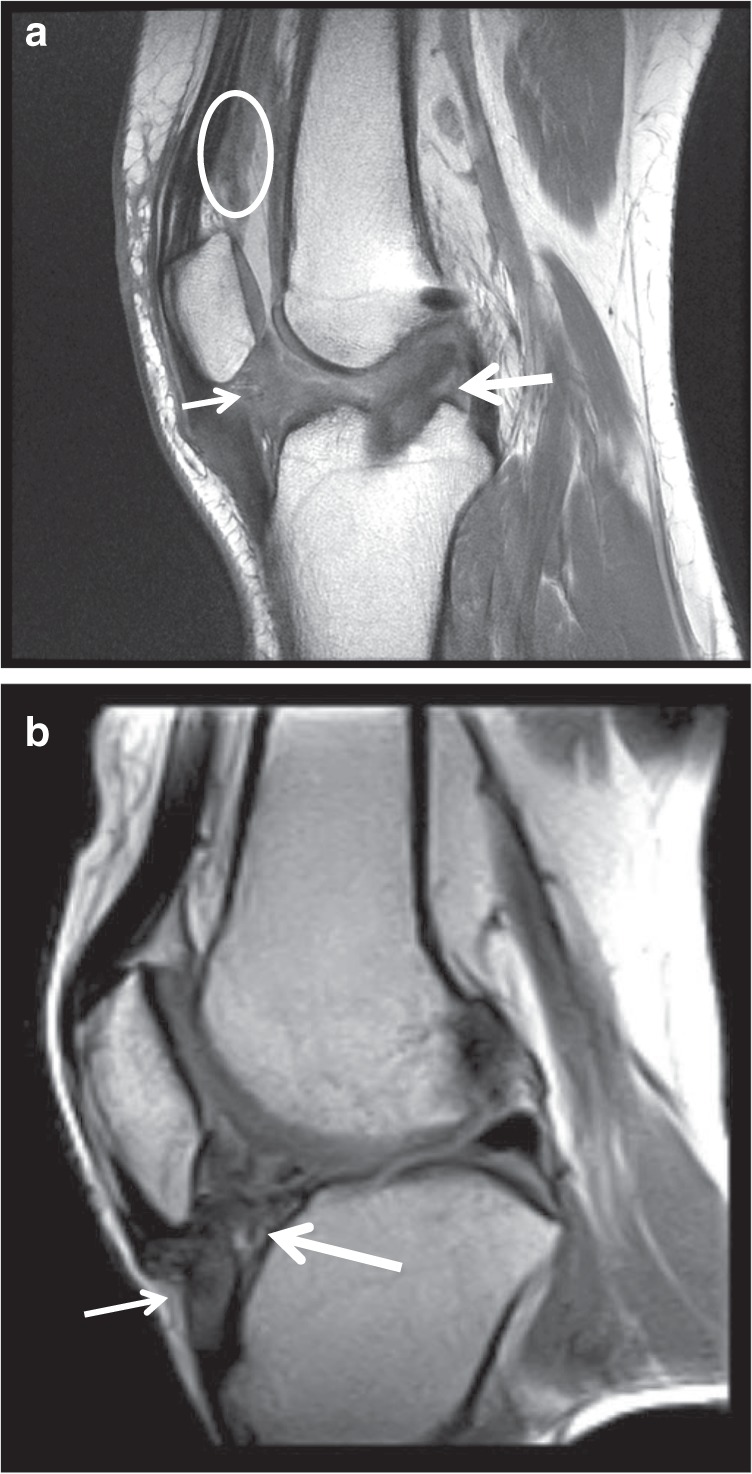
a Sagittal fast spin echo intermediate-weighted image of a 33-year-old woman with clinical stiffness following anterior cruciate ligament (ACL) reconstruction, showing scarring of the synovium around the ACL reconstruction (long arrow) as well as the central portion of the deep infrapatellar fat pad (short arrow) and the lining of the suprapatellar recess (oval). b Magnetic resonance imaging of the knee of a 49-year-old male with clinical stiffness 2 months following a meniscus operation, showing deep infrapatellar fat pad scarring (long arrow) and shortening of the patella tendon (short arrow) with resultant patella infera (abnormally low lying patella)
